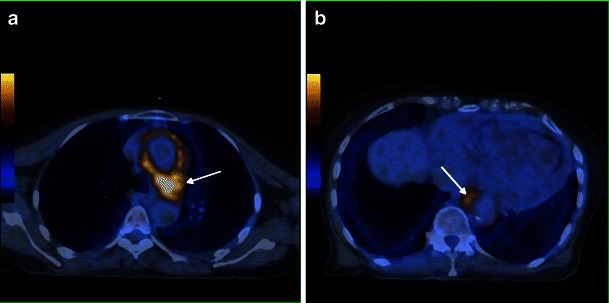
Axial FDG-PET/CT fusion images in a 71-year-old female, in the upper (a) and mid (b) portion of the thorax, showing elevated metabolic activity involving the aortic arch (arrow) and descending aorta (arrow)
